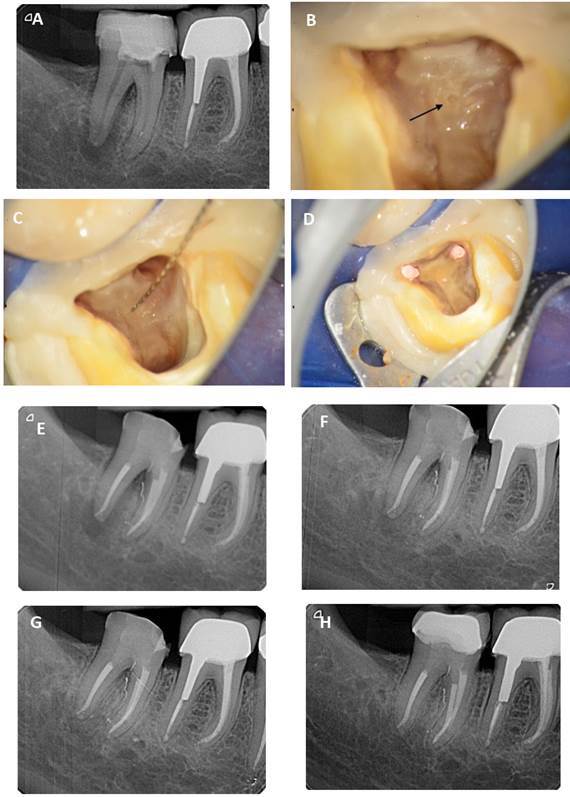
(A) Preoperative intraoral X-Ray focused on tooth #47; (B)
